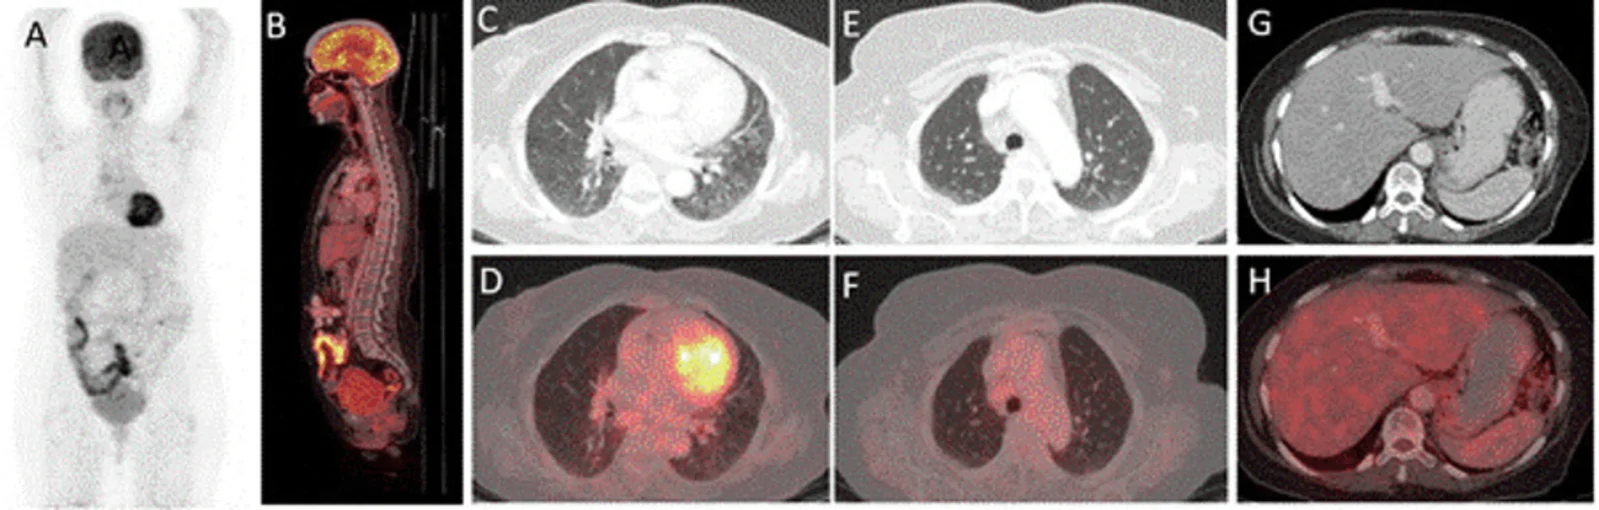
Post-treatment ^18F-FDG PET/CT demonstrating complete metabolic response after temozolomide and rucaparib Post-treatment ^18F-FDG PET/CT obtained after three cycles of temozolomide and rucaparib. (A) Maximum intensity projection (MIP) image showing complete resolution of the previously noted foci of increased FDG uptake in the right hemithorax. (B) Sagittal fused PET/CT image demonstrating post-hysterectomy status without evidence of FDG-avid local residual or recurrent disease. (C, E) Axial CT images and (D, F) corresponding fused PET/CT images showing complete resolution of the previously identified FDG-avid metastatic right lung nodules. (G) Axial CT image and (H) corresponding fused PET/CT image demonstrating complete resolution of the previously noted right pleural effusion. Overall findings are consistent with a complete metabolic response. FDG: fluorodeoxyglucose, PET/CT: positron emission tomography/computed tomography
